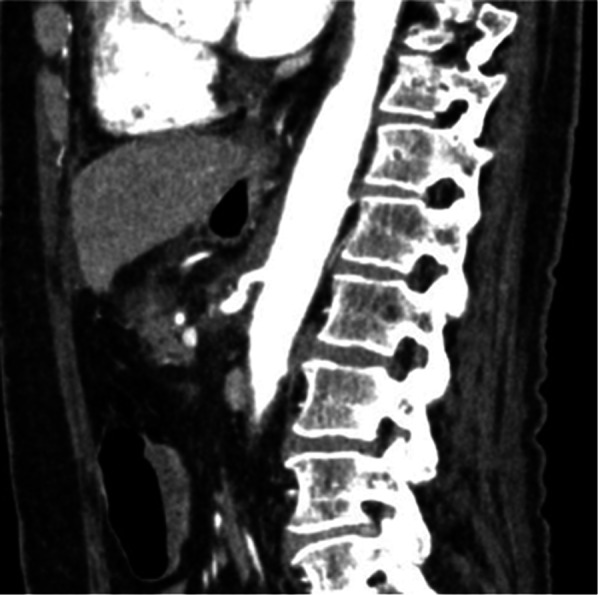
Abdominal contrast-enhanced computed tomography on postoperative day 7. Sagittal image demonstrating improved celiac artery patency
